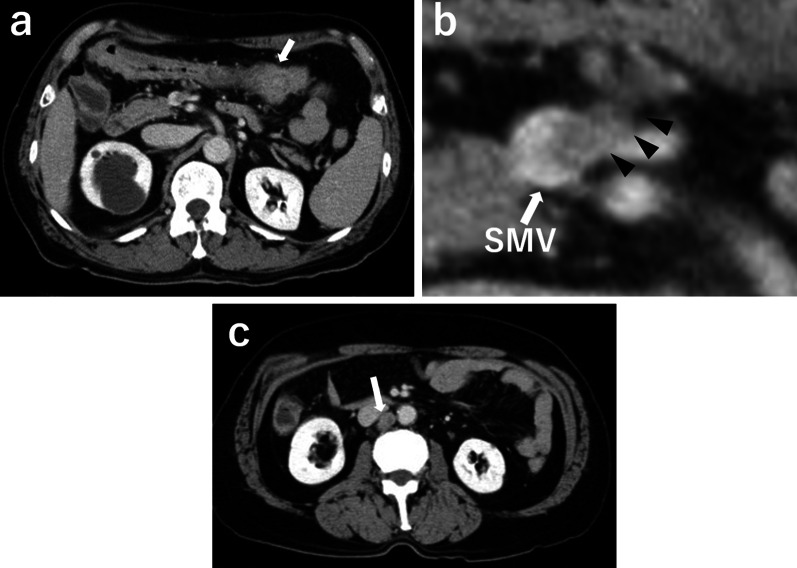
Computed tomography (CT) images. Abdominal CT showing an enhanced mass in the transverse colon (a, arrow), with mesenteric lymph node metastasis invading the superior mesenteric vein (b enlarged image of a, arrowhead) and paraaortic lymph node metastasis (c, arrow). SMV, superior mesenteric vein
